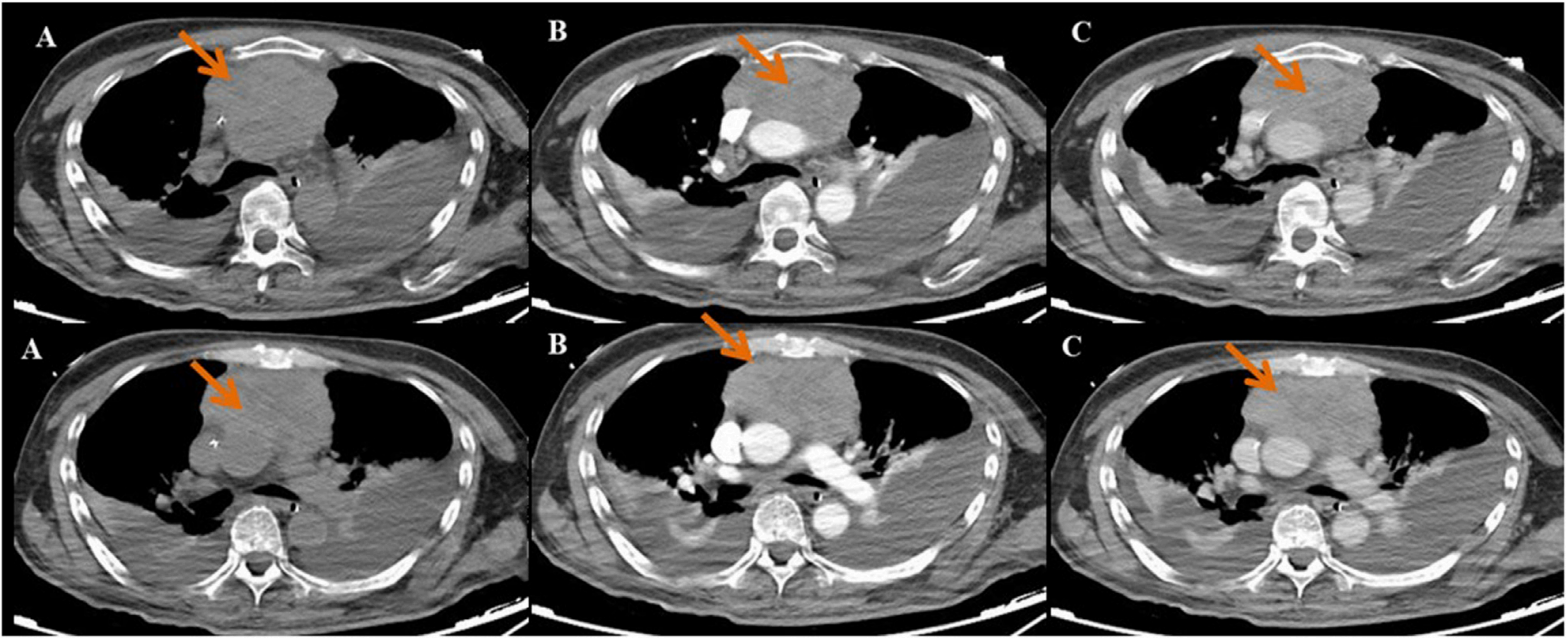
The enhanced chest CT indicated that the size of the huge thymoma is 8.7 × 9.0 cm (red arrow). (A) Plain scan sequence; (B) arterial phase; and (C) venous phase.
